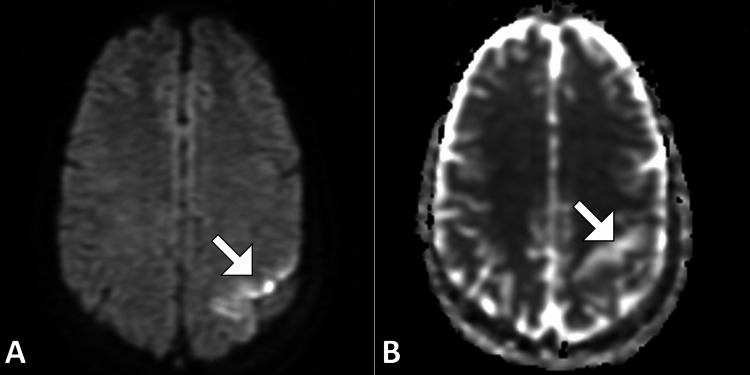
DWI and ADC mapping of the left parietal lobe. DWI (A) shows no areas of true restricted diffusion in the lesion. The corresponding ADC map (B) demonstrates facilitated diffusion in the same region (white arrow), consistent with vasogenic edema rather than cytotoxic edema. These findings help differentiate thrombotic edema from acute infarction. DWI = diffusion-weighted imaging; ADC = apparent diffusion coefficient
